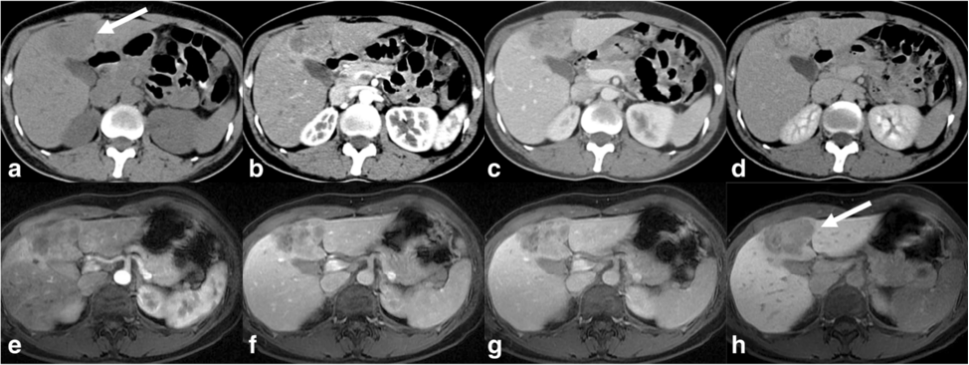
CT and MRI appearances of mass forming type of iCCA. Top row: non contrast enhanced (a), post contrast enhanced arterial phase (b), portal venous phase (c) and delayed phase (d) CT images. Bottom row: post contrast enhanced MRI images in arterial phase (e), portal venous phase (f), delayed phase (g), and 20-minute delay post Gd-EOB-DTPA image (h). The mass is iso- to hypodense to liver and shows similar contrast enhancement characteristics on both CT and MRI with peripheral rim like arterial phase enhancement with centripetal enhancement in the portal venous and delayed phases without any washout. In the hepatobiliary phase there is no uptake of Gd-EOB-DTPA by the mass suggesting a non-hepatocellular tumor.
